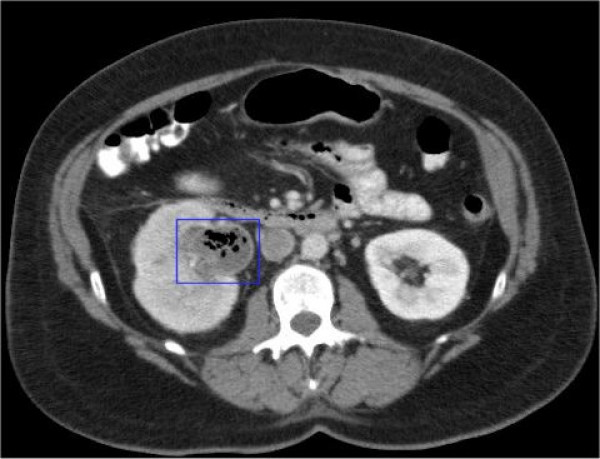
CT scan showing a moderate hydronephrosis, with gas in a dilated pelvicalyceal system.
